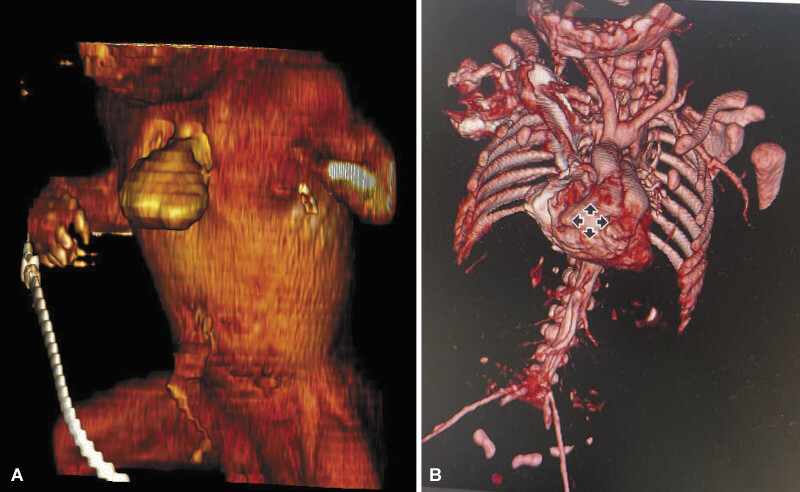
Three-dimensional CT reconstructions of complete thoracic ectopia cordis. (
